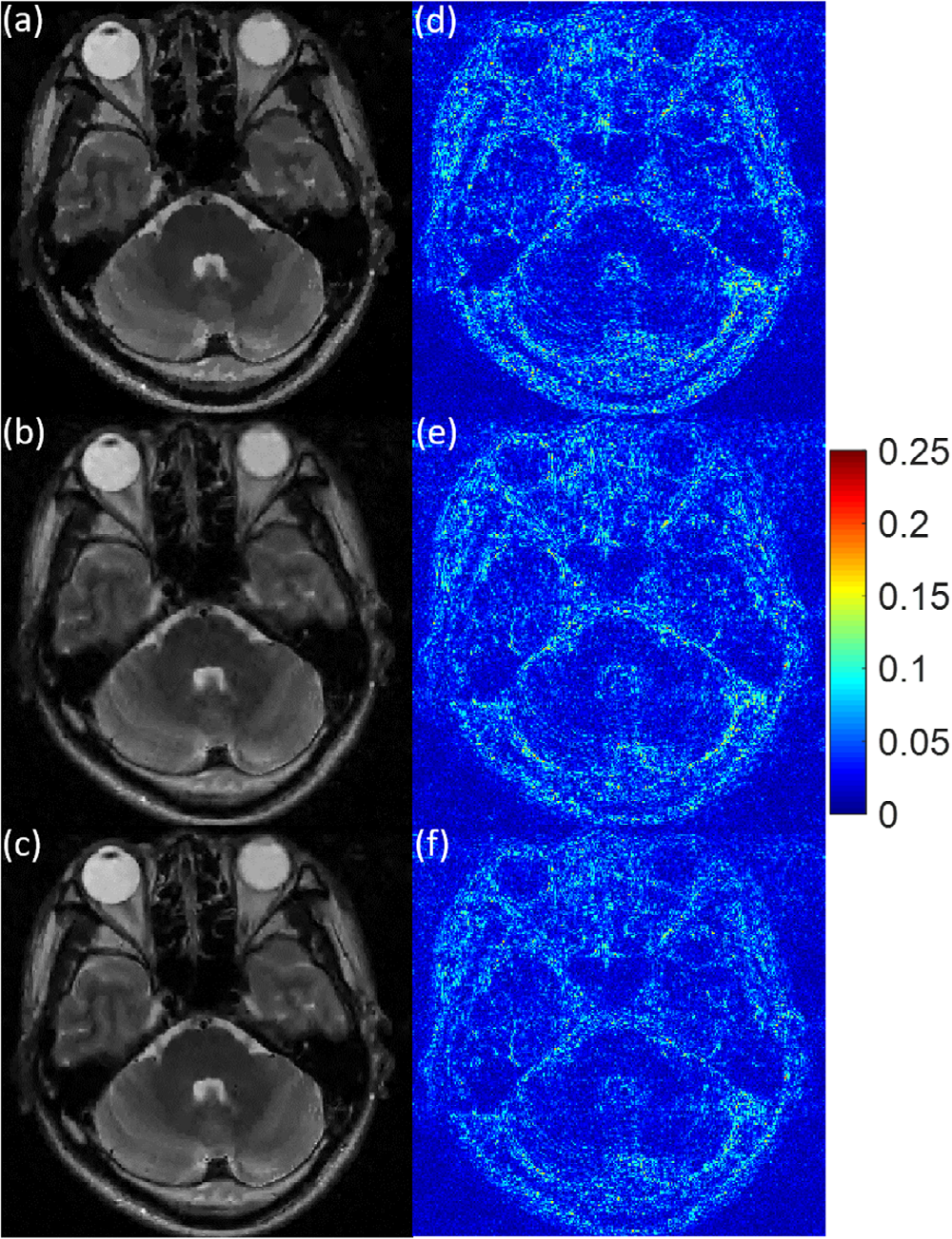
Reconstruction results of the 31st slice of the brain MRI 2. The parts of the figure (a)–(c) are the reconstruction results of MCTV‐L_2_, pFISTA, and TV+TFL_0_. The parts (d)–(f) are corresponding error images of (a)–(c).
